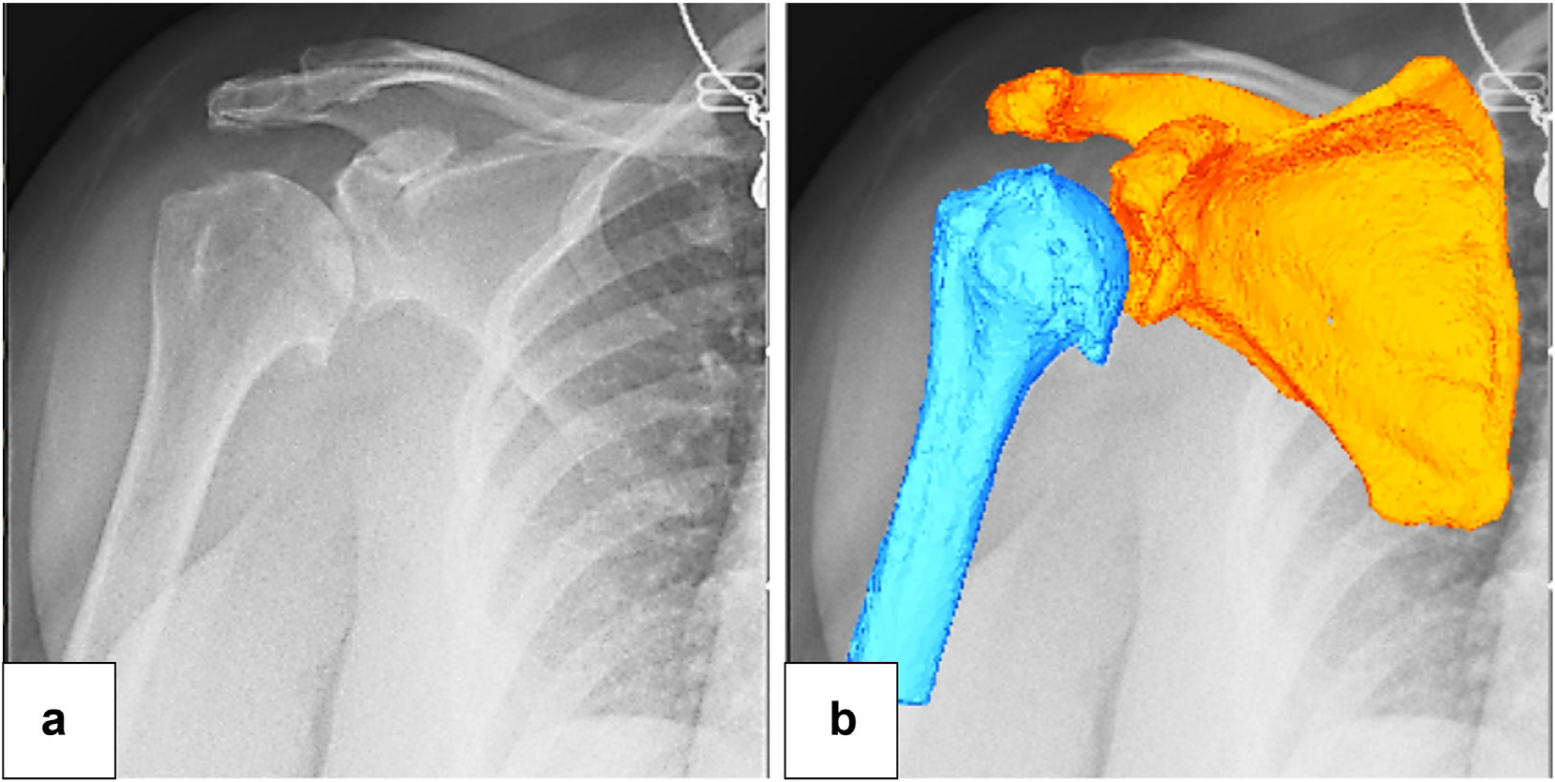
The three-dimensional to 2-dimensional model image registration for reverse total shoulder arthroplasty. (a) A DDR image during scapular plane abduction. (b) Three-dimensional models were matched with the silhouette of the implants on the DDR image. *DDR*, dynamic digital radiography.
